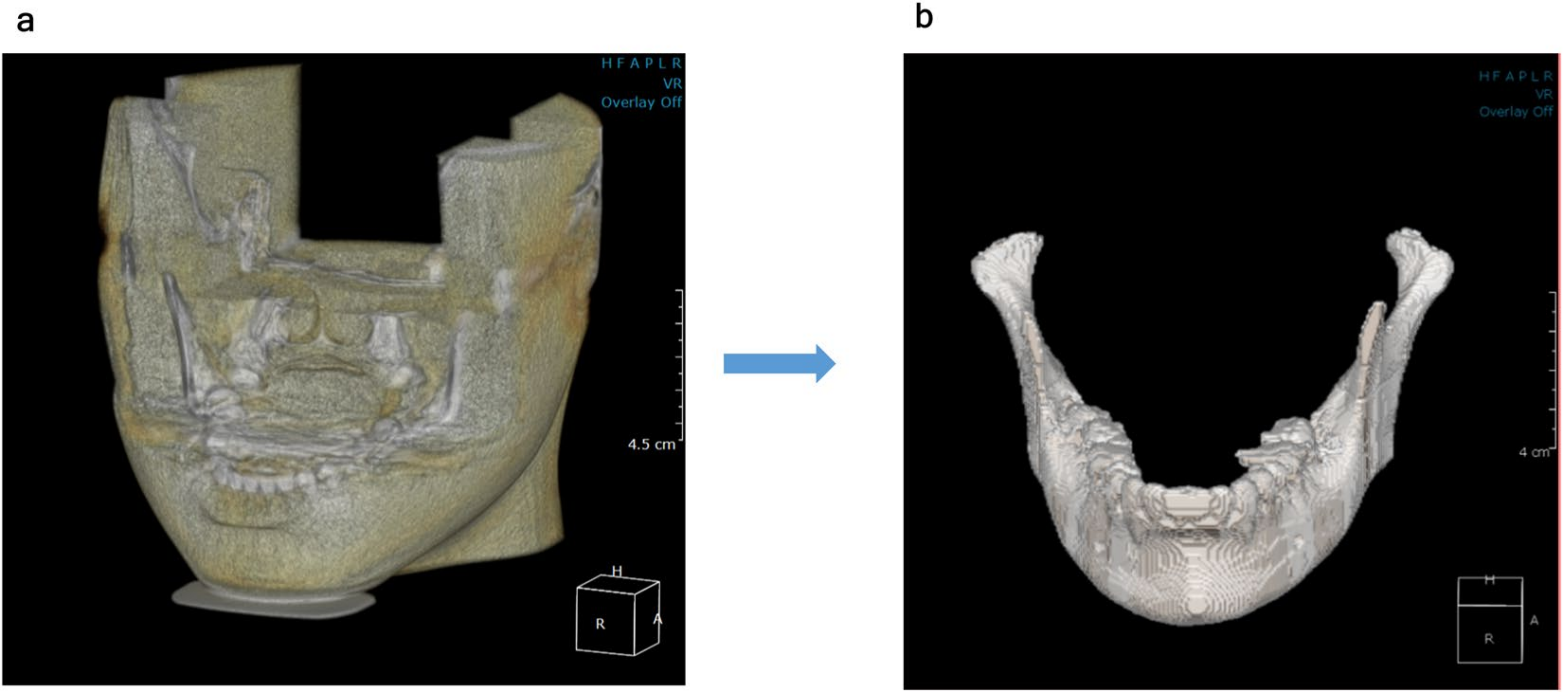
Binarization of the mandible. (a) Representation of the processed 3D CBCT image. (b) The 3D images were binarized using Otsu’s method and the cumulative binarization algorithm until reaching the upper points of the condylar head obtained in the previous step of removing the upper portion of the bilateral condylar heads (Fig. 3d). 3D, 3-dimensional; CBCT, cone-beam computed tomography.
